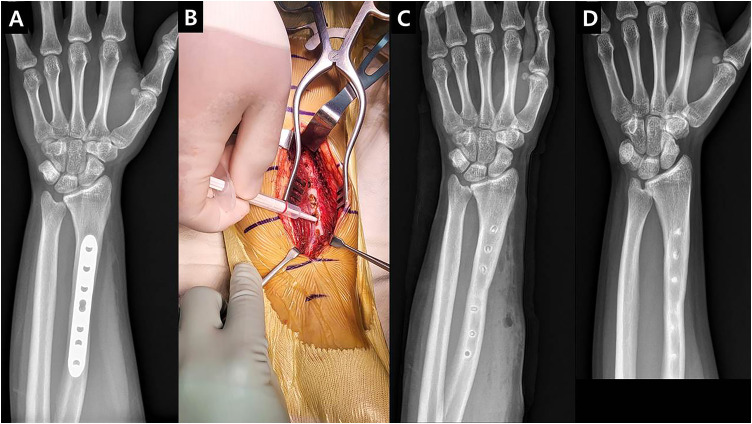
Adult radius implant removal case. A: 21-year-old patient underwent Compressive Plating fixation using LC-DCP after a distal radius corrective osteotomy for 1 year. The osteotomy site was judged completely healed. B: Implant removal and scar revision were performed simultaneously; curettage was performed around the screw hole, followed by insertion of a syringe-type pure β-TCP (Neobone^®^) bone substitute graft. C: Immediately following implant removal surgery, an X-ray reveals the grafted pure β-TCP (Neobone^®^) bone substitute positioned within the hole. D: 1 year after the final surgery, the X-ray shows that the grafted pure β-TCP (Neobone^®^) bone substitute has contributed to host bone formation and achieved cortical healing.
